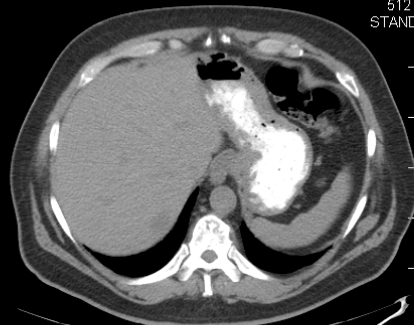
Computed tomography scan of abdomen, showing multiple hypodense nodules in the liver.
